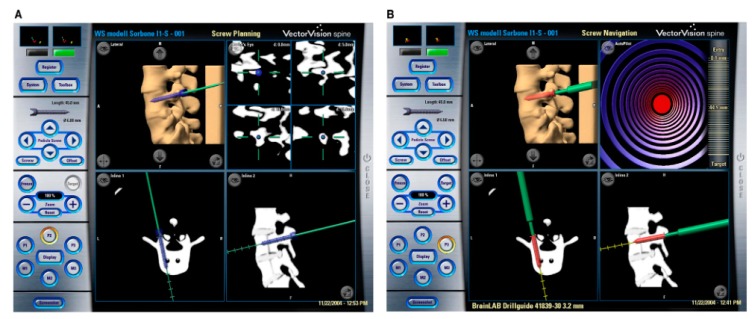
CT-based navigation feedback within the pre-operative planning (a) and (b) reports intra-operative navigation of the pedicle screw placement [3].
